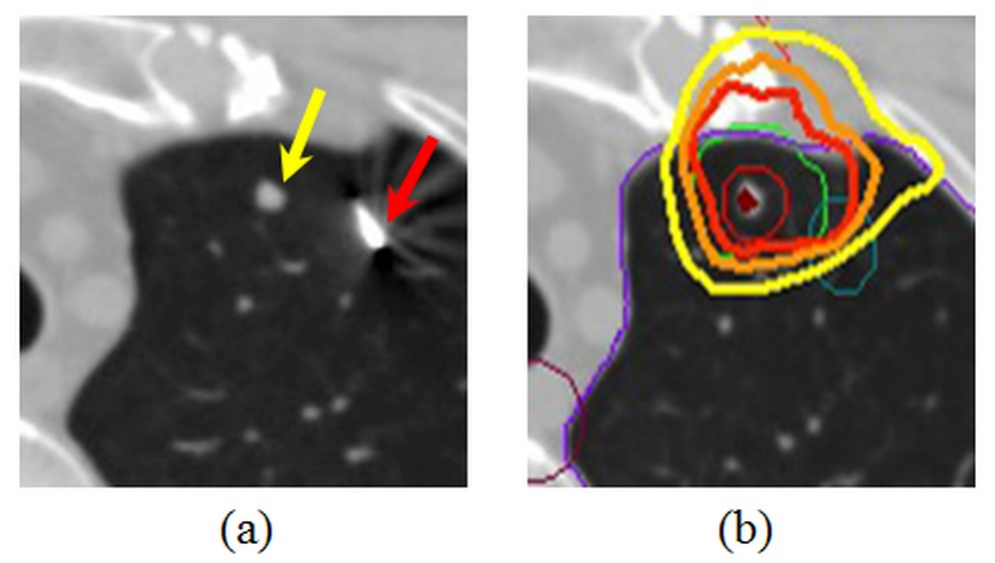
Computed tomography images (a) The yellow arrow shows a tumor whereas the red arrow is a fiducial marker. (b) The thick red, orange, and yellow lines are isodose contours of 3.0, 2.8, and 2.6 Gy/fraction, respectively. The thin red, green, and blue lines represent the clinical target volume, the planning target volume with an isotropic margin of 5 mm, and the marker regions, respectively.
